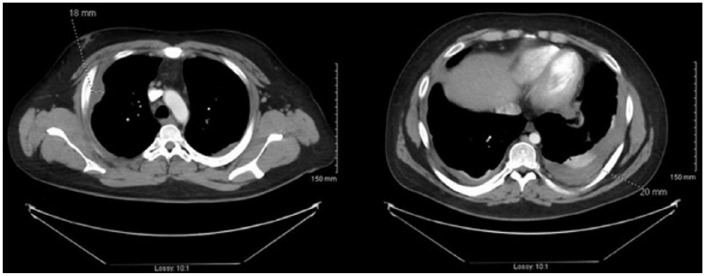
Computed tomography scan demonstrating pleural opacities and thickening.
